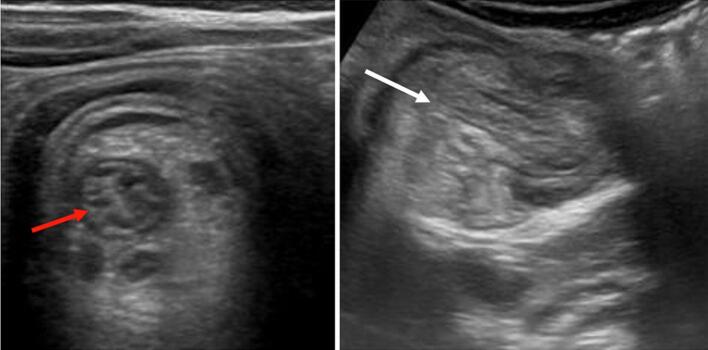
Abdominal ultrasound showing a roundel image (red arrow) and sandwich image (white arrow) showing intestinal telescoping. (For interpretation of the references to colour in this figure legend, the reader is referred to the web version of this article.)
